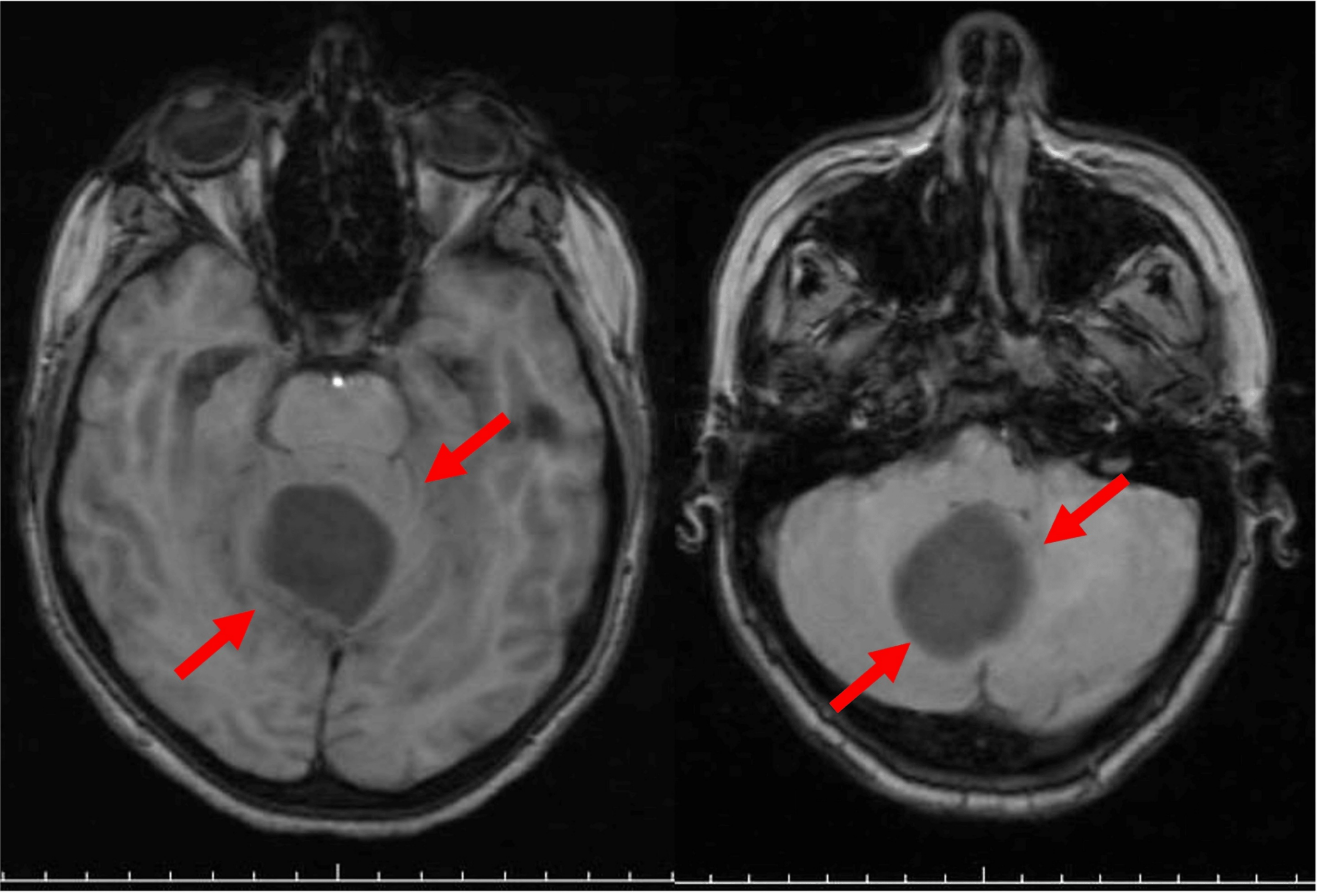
Brain MRI: note the hypointense nodule centered in the IV ventricle, shaping the cerebellar parenchyma (red arrows).
